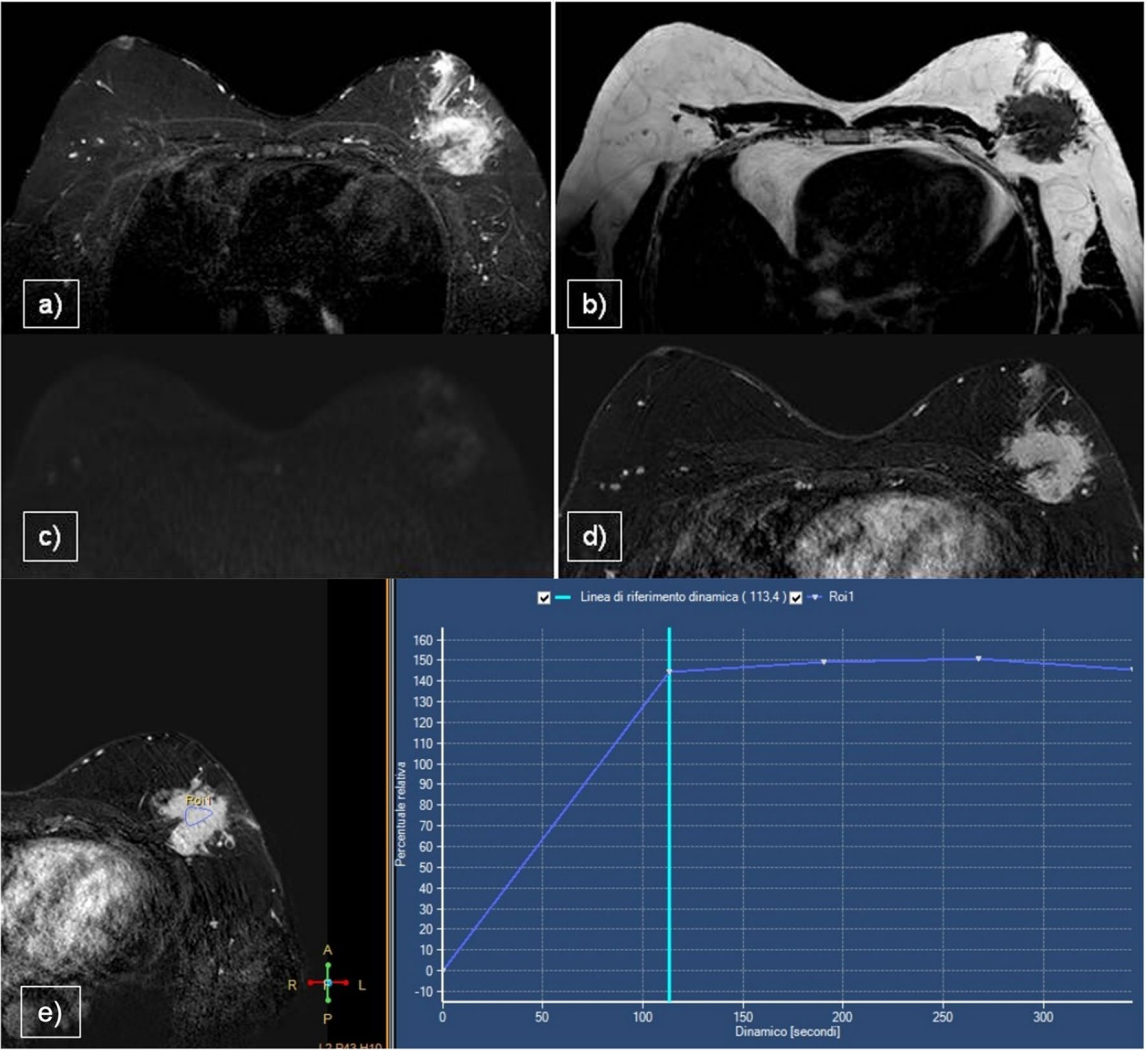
a STIR, b T2WI, c DWI, d CE T1WI, e Time–Intensity Curve. A 6-cm hard lesion is observed on the left breast, consisting of two different tissue components: a hyperintense stromal component in STIR and a hypointense fibrous component in all sequences (a,b). The mass demonstrates low-signal restriction in DWI (c), intense ehancement (d) and a plateau time–intensity curve (e)
